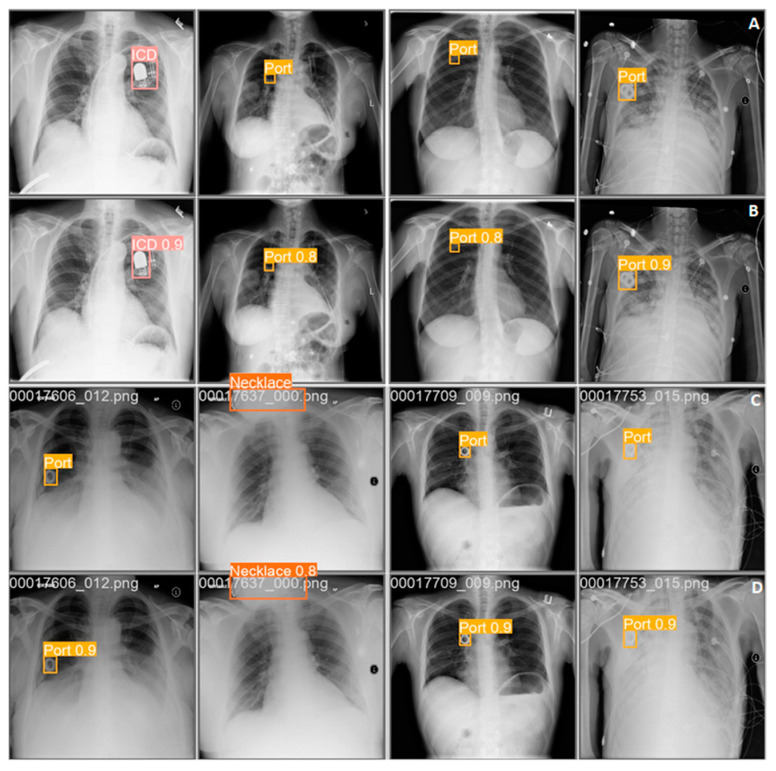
The image shows a comparison of the placement of bounding boxes (rectangular-shaped and square-shaped areas in pink, yellow, and orange colours) labeled by the model and the annotator evaluating the X-ray images. Rows A and C represent images evaluated by the annotator, whereas rows B and D were labeled by the AI.
